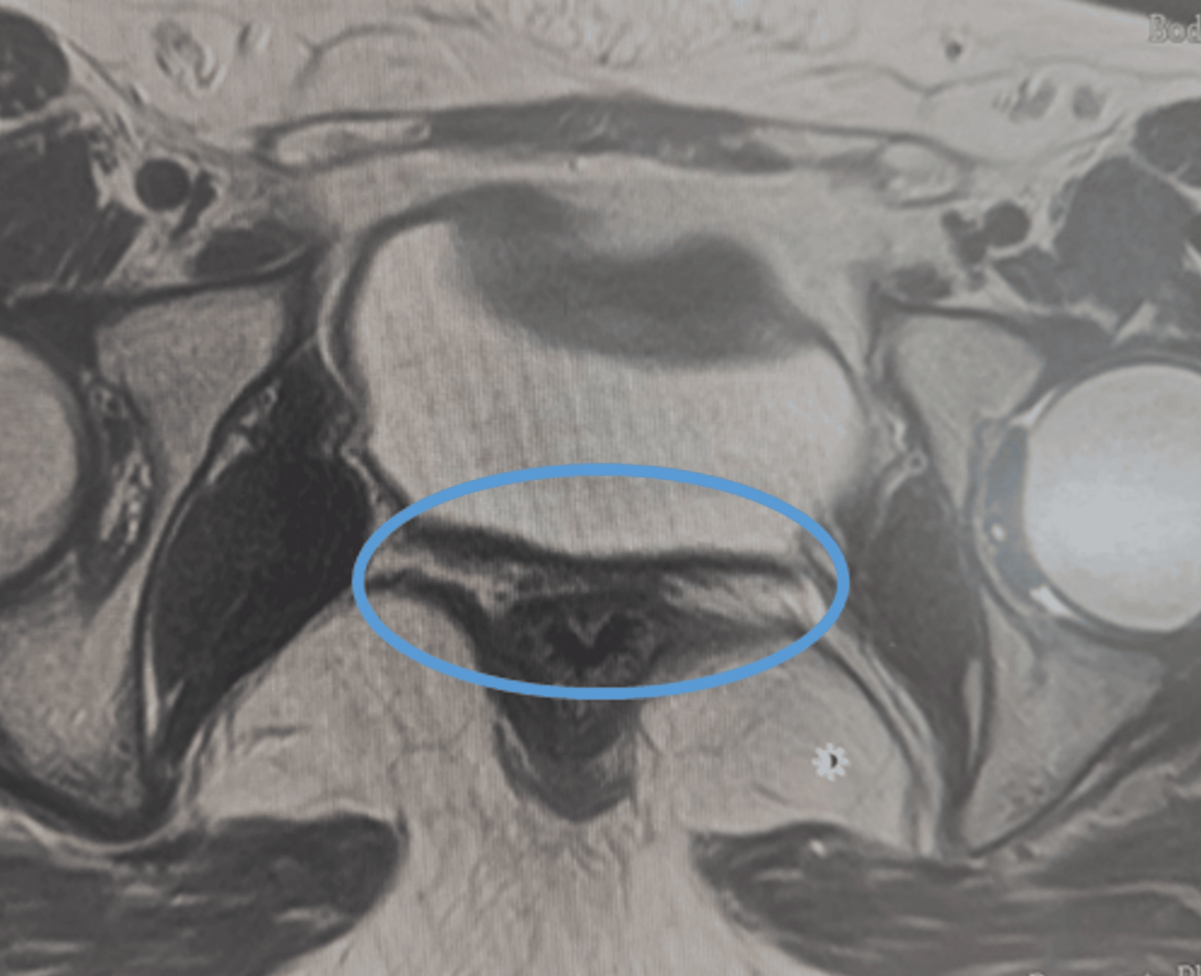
Pelvic magnetic resonance imaging: axial section. The circled area highlights the location where the uterus is absent.
